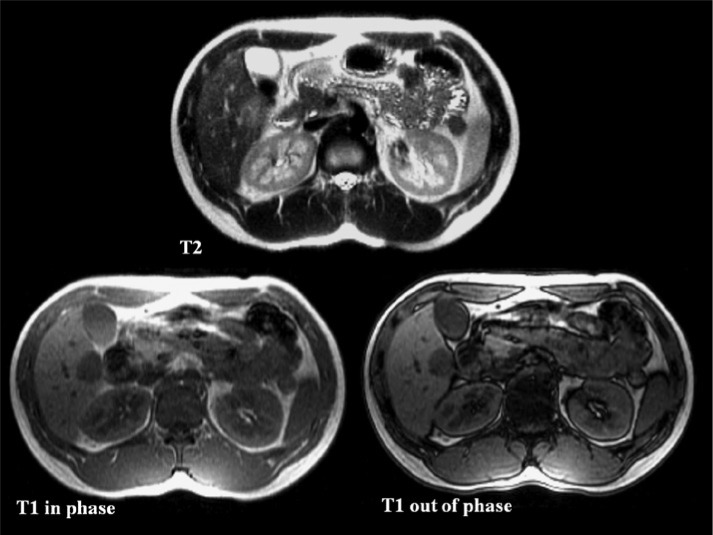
Gadoxetic acid (Gd-EOB-DTPA)–enhanced magnetic resonance images. T2-weighted axial imaging (upper) shows the following: well-circumscribed hepatic nodule, central very high signal intensity with intermediate high signal in the periphery. T1-weighted in-phase (lower left)/out-of- phase (lower right) axial shows the following: low signal intensity without signal drop of fat.
